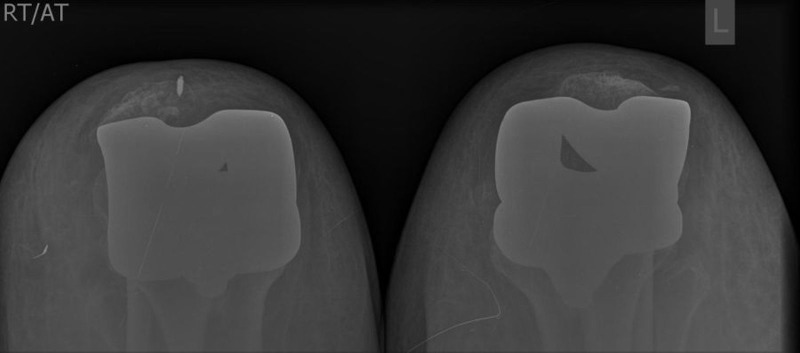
Postoperative follow-up x-ray with skyline view both knees showing normal position of the patellas.
